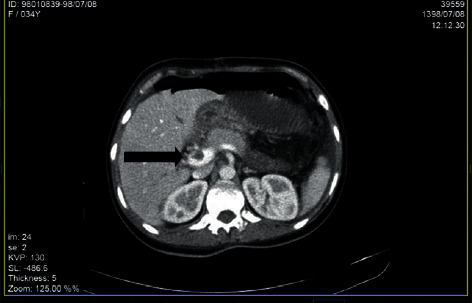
Thrombosis in main portal vein in spiral abdominal CT scan with IV contrast (black arrow).
